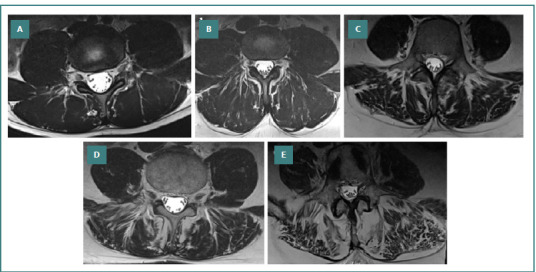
The Goutallier classification of overall qualitative fatty infiltration of the paraspinal muscles was exemplified in five patients from our cohort on axial T2-weighted sequences. A, grade 0, no visible fatty infiltration of the paraspinal muscles; B, grade 1, a few fatty streaks are visible within the paraspinal muscles; C, grade 2, less than 50% of paraspinal muscle atrophied; D, grade 3, an equal amount of muscle and fat is present within the paraspinal muscles; E, grade 4, more than 50% of the paraspinal muscle is fatty infiltration.
